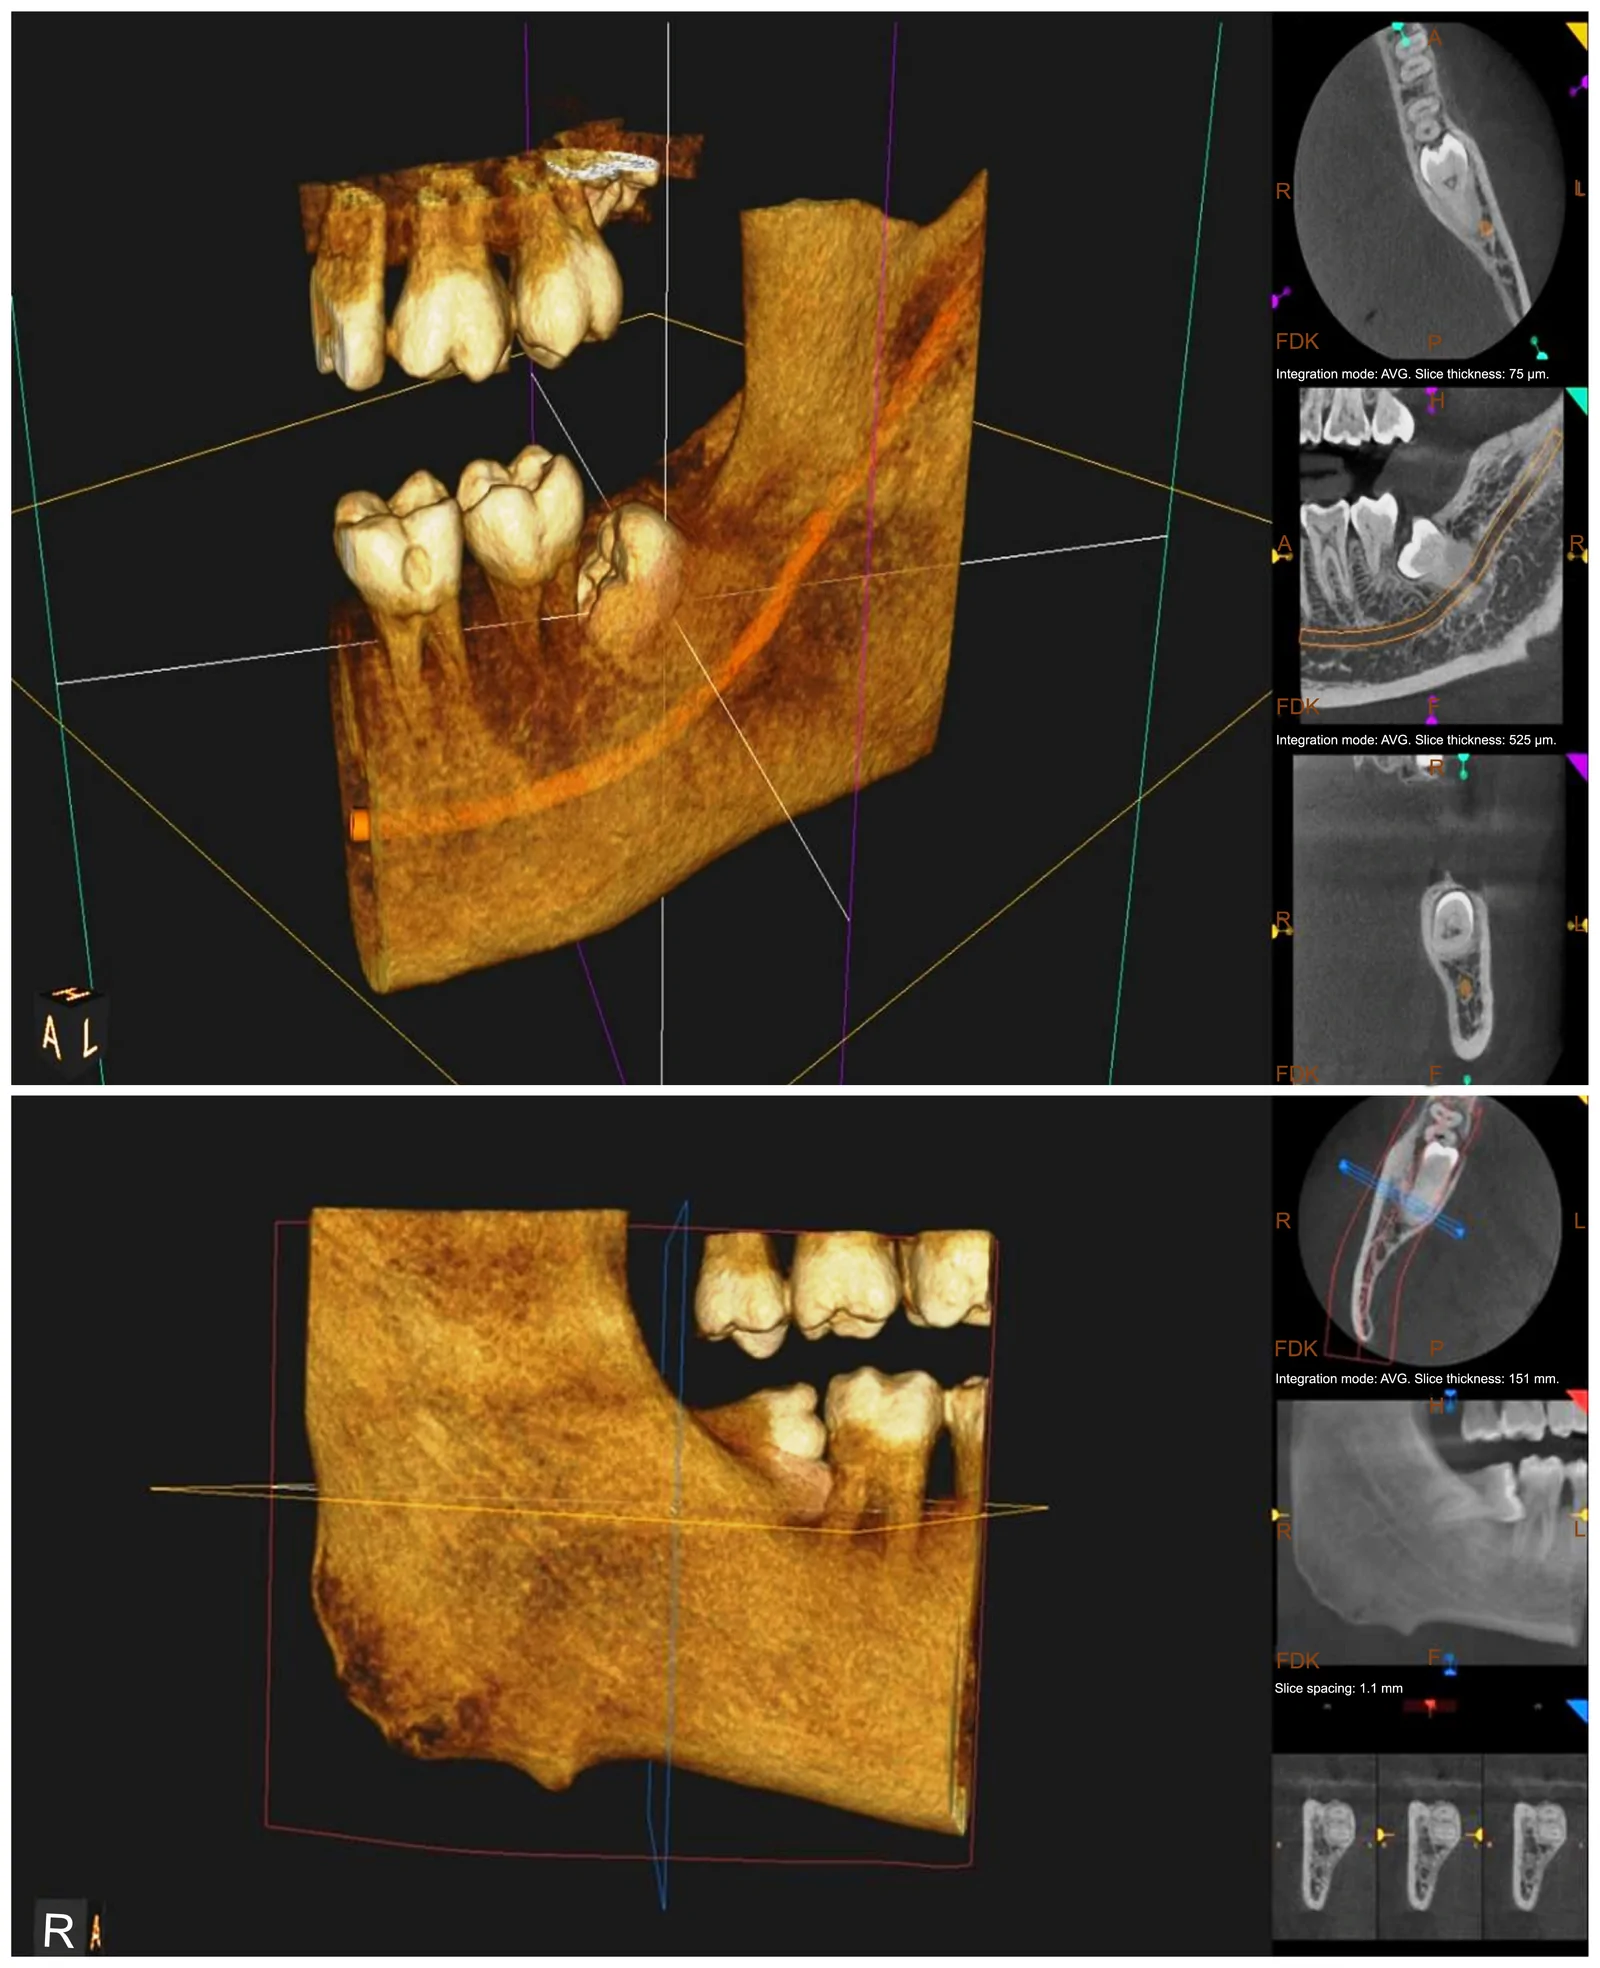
CBCT views of impacted mandibular third molar.
